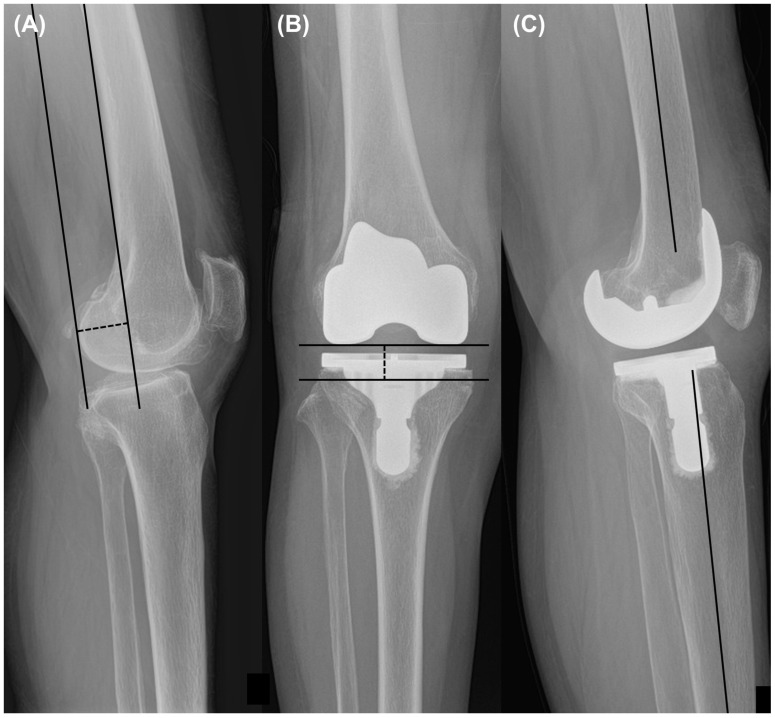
Measurement of (A) femoral posterior condylar offset, (B) joint line distance on anteroposterior radiographs, and (C) extension angle on full extension lateral view radiographs.
